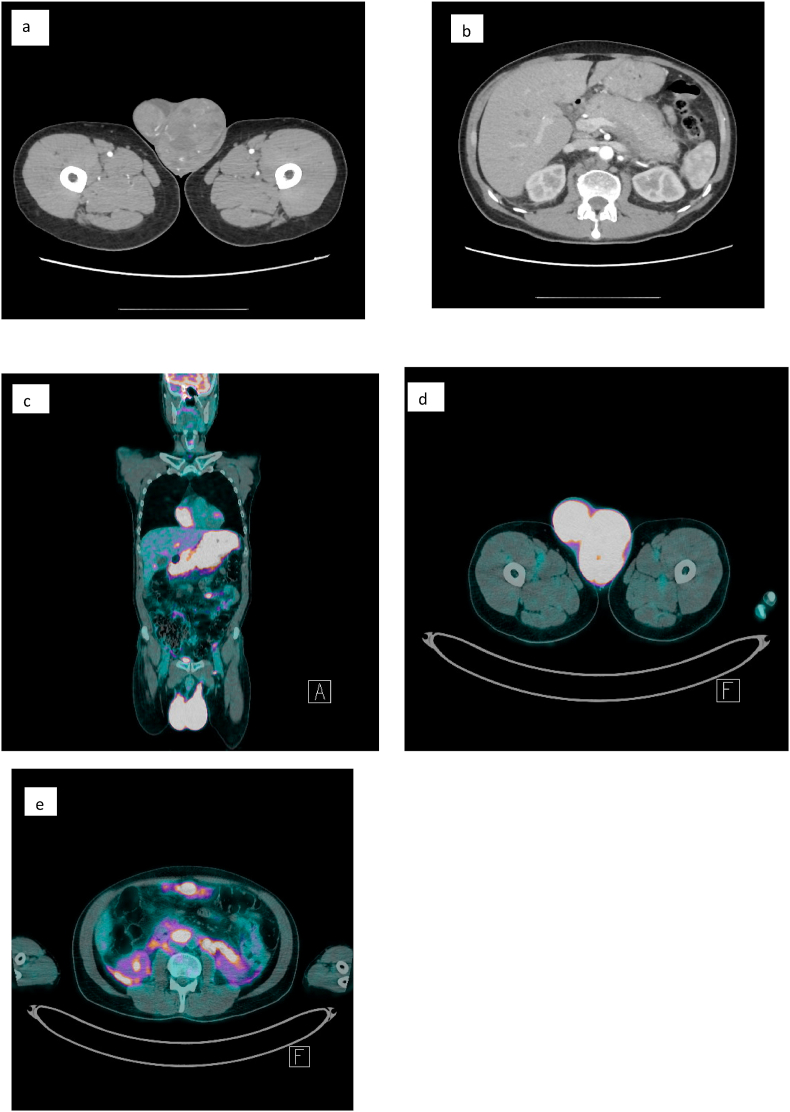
(A–b) CT abdomen - pelvis with and without contrast. Numerous supracentimetric lymphadenopathies were evident in the abdominal and At the level of the scrotum, markedly increased didymes bilaterally were highlighted with a strongly inhomogeneous appearance and with hypodense gaps in the context; the left didymus was more enlarged and more inhomogeneous and shows axial diameters of 92 × 50 mm. (c–e) 18F- FDG PET: The presence of pathological hyperaccumulation of the radiopharmaceutical Was present in all body districts examined.
